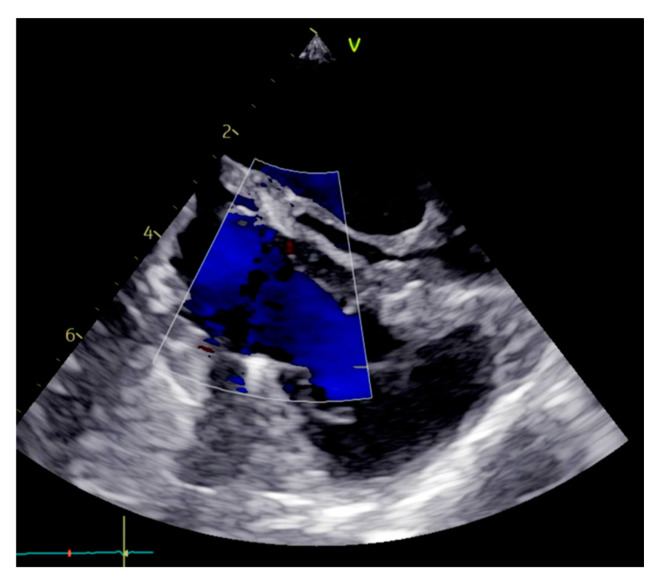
Post-operative transesophageal echocardiogram.
